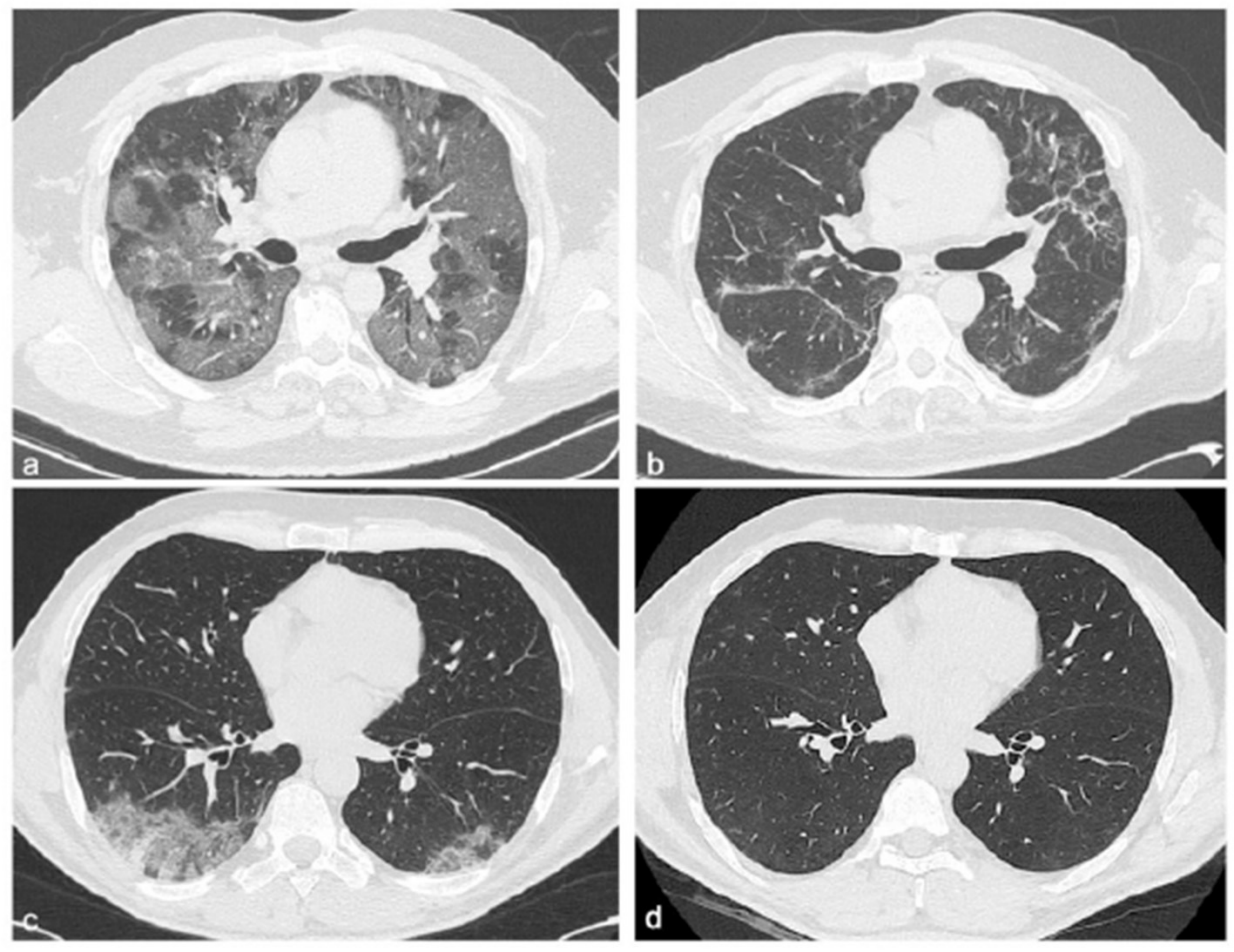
Chest CT features of two patients with COVID-19 pneumonia at different time points: hospitalization and 6 months after discharge. Chest CT images of a 58-year-old male patient with COVID-19, not recovery patient (a,b). The first CT performed at admission shows bilateral areas of ground-glass opacities in a peripheral distribution (a), and after 6 months from discharge, CT shows persistent of interlobular septal thickening with peripheral distribution (b). Chest CT images of a 51-year-old male patient with COVID-19, recovery patient (c,d). The first CT shows, at admission, a small consolidation at the right lower lobe accompanied by ground-glass opacities in both lower lobes (c), and after 6 months from discharge, no residual abnormalities were observed (d).
